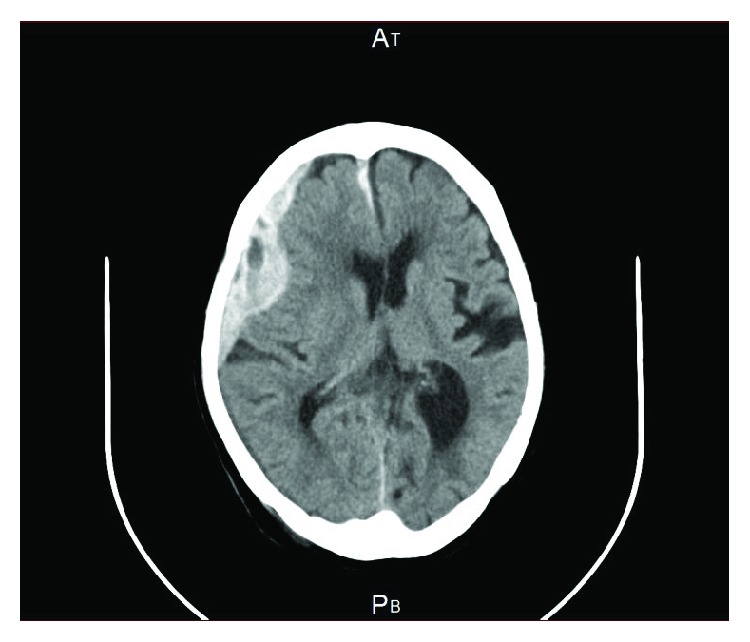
Magnetic resonance imaging of the horizontal section at the frontal lobe of the cerebrum.
